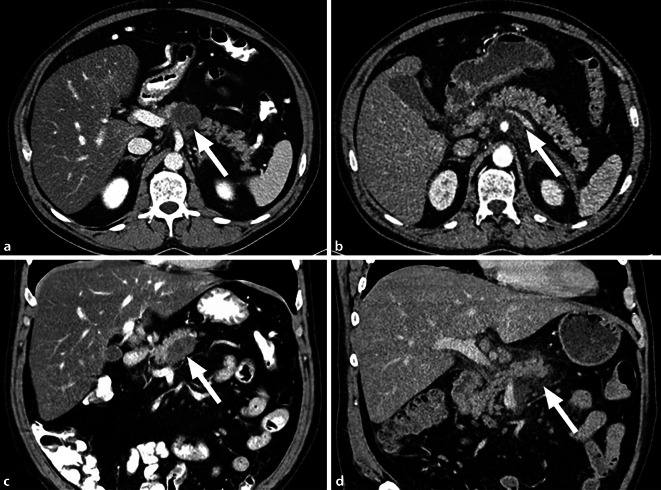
CT-Abdomen: suspekter Pankreaskopftumor. a, b Zeitpunkt des erhobenen V. a. Raumforderung. c, d Trauma-CT zum Unfallzeitpunkt ein Jahr zuvor. Jeweils in koronarer und transversaler Ansicht
